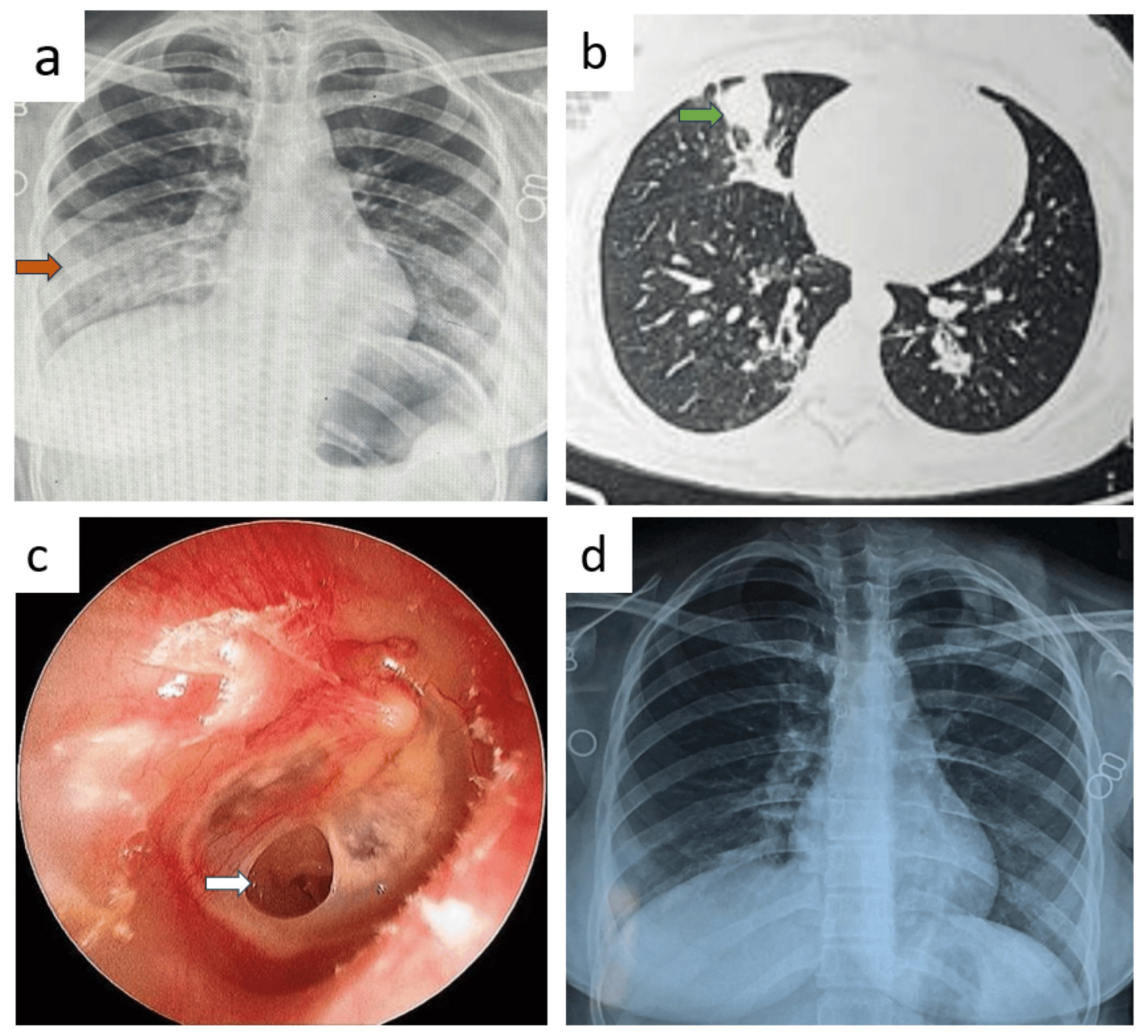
Radiology and otoscopy images of patient F before and after treatment. (a) Imaging studies demonstrated a non-homogenous opacity in the right lower lung zone on chest X-ray marked by a brown arrow. (b) CT showed right middle lobe consolidation marked by a green arrow. (c) Otoscopic image of the tympanic membrane of right ear showing perforation in the anteroinferior quadrant with smooth edges suggestive of chronic otitis media marked by a white arrow. (d) After a treatment period of three months, follow-up imaging showed complete resolution of the opacities.
